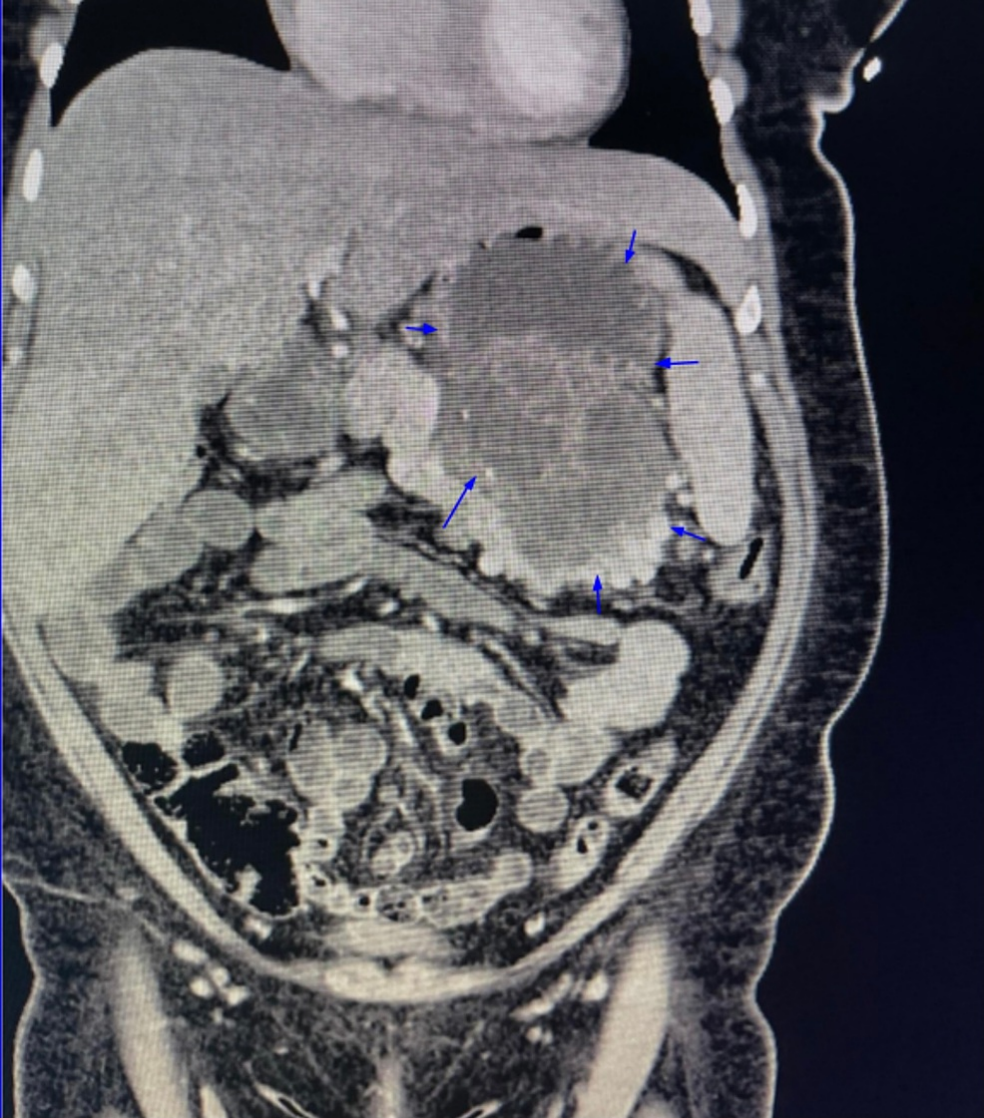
Non-contrast CT scan of the abdomen in a coronal plane three months post-surgery. Blue arrows highlight the boundaries of the pancreatic cyst.
